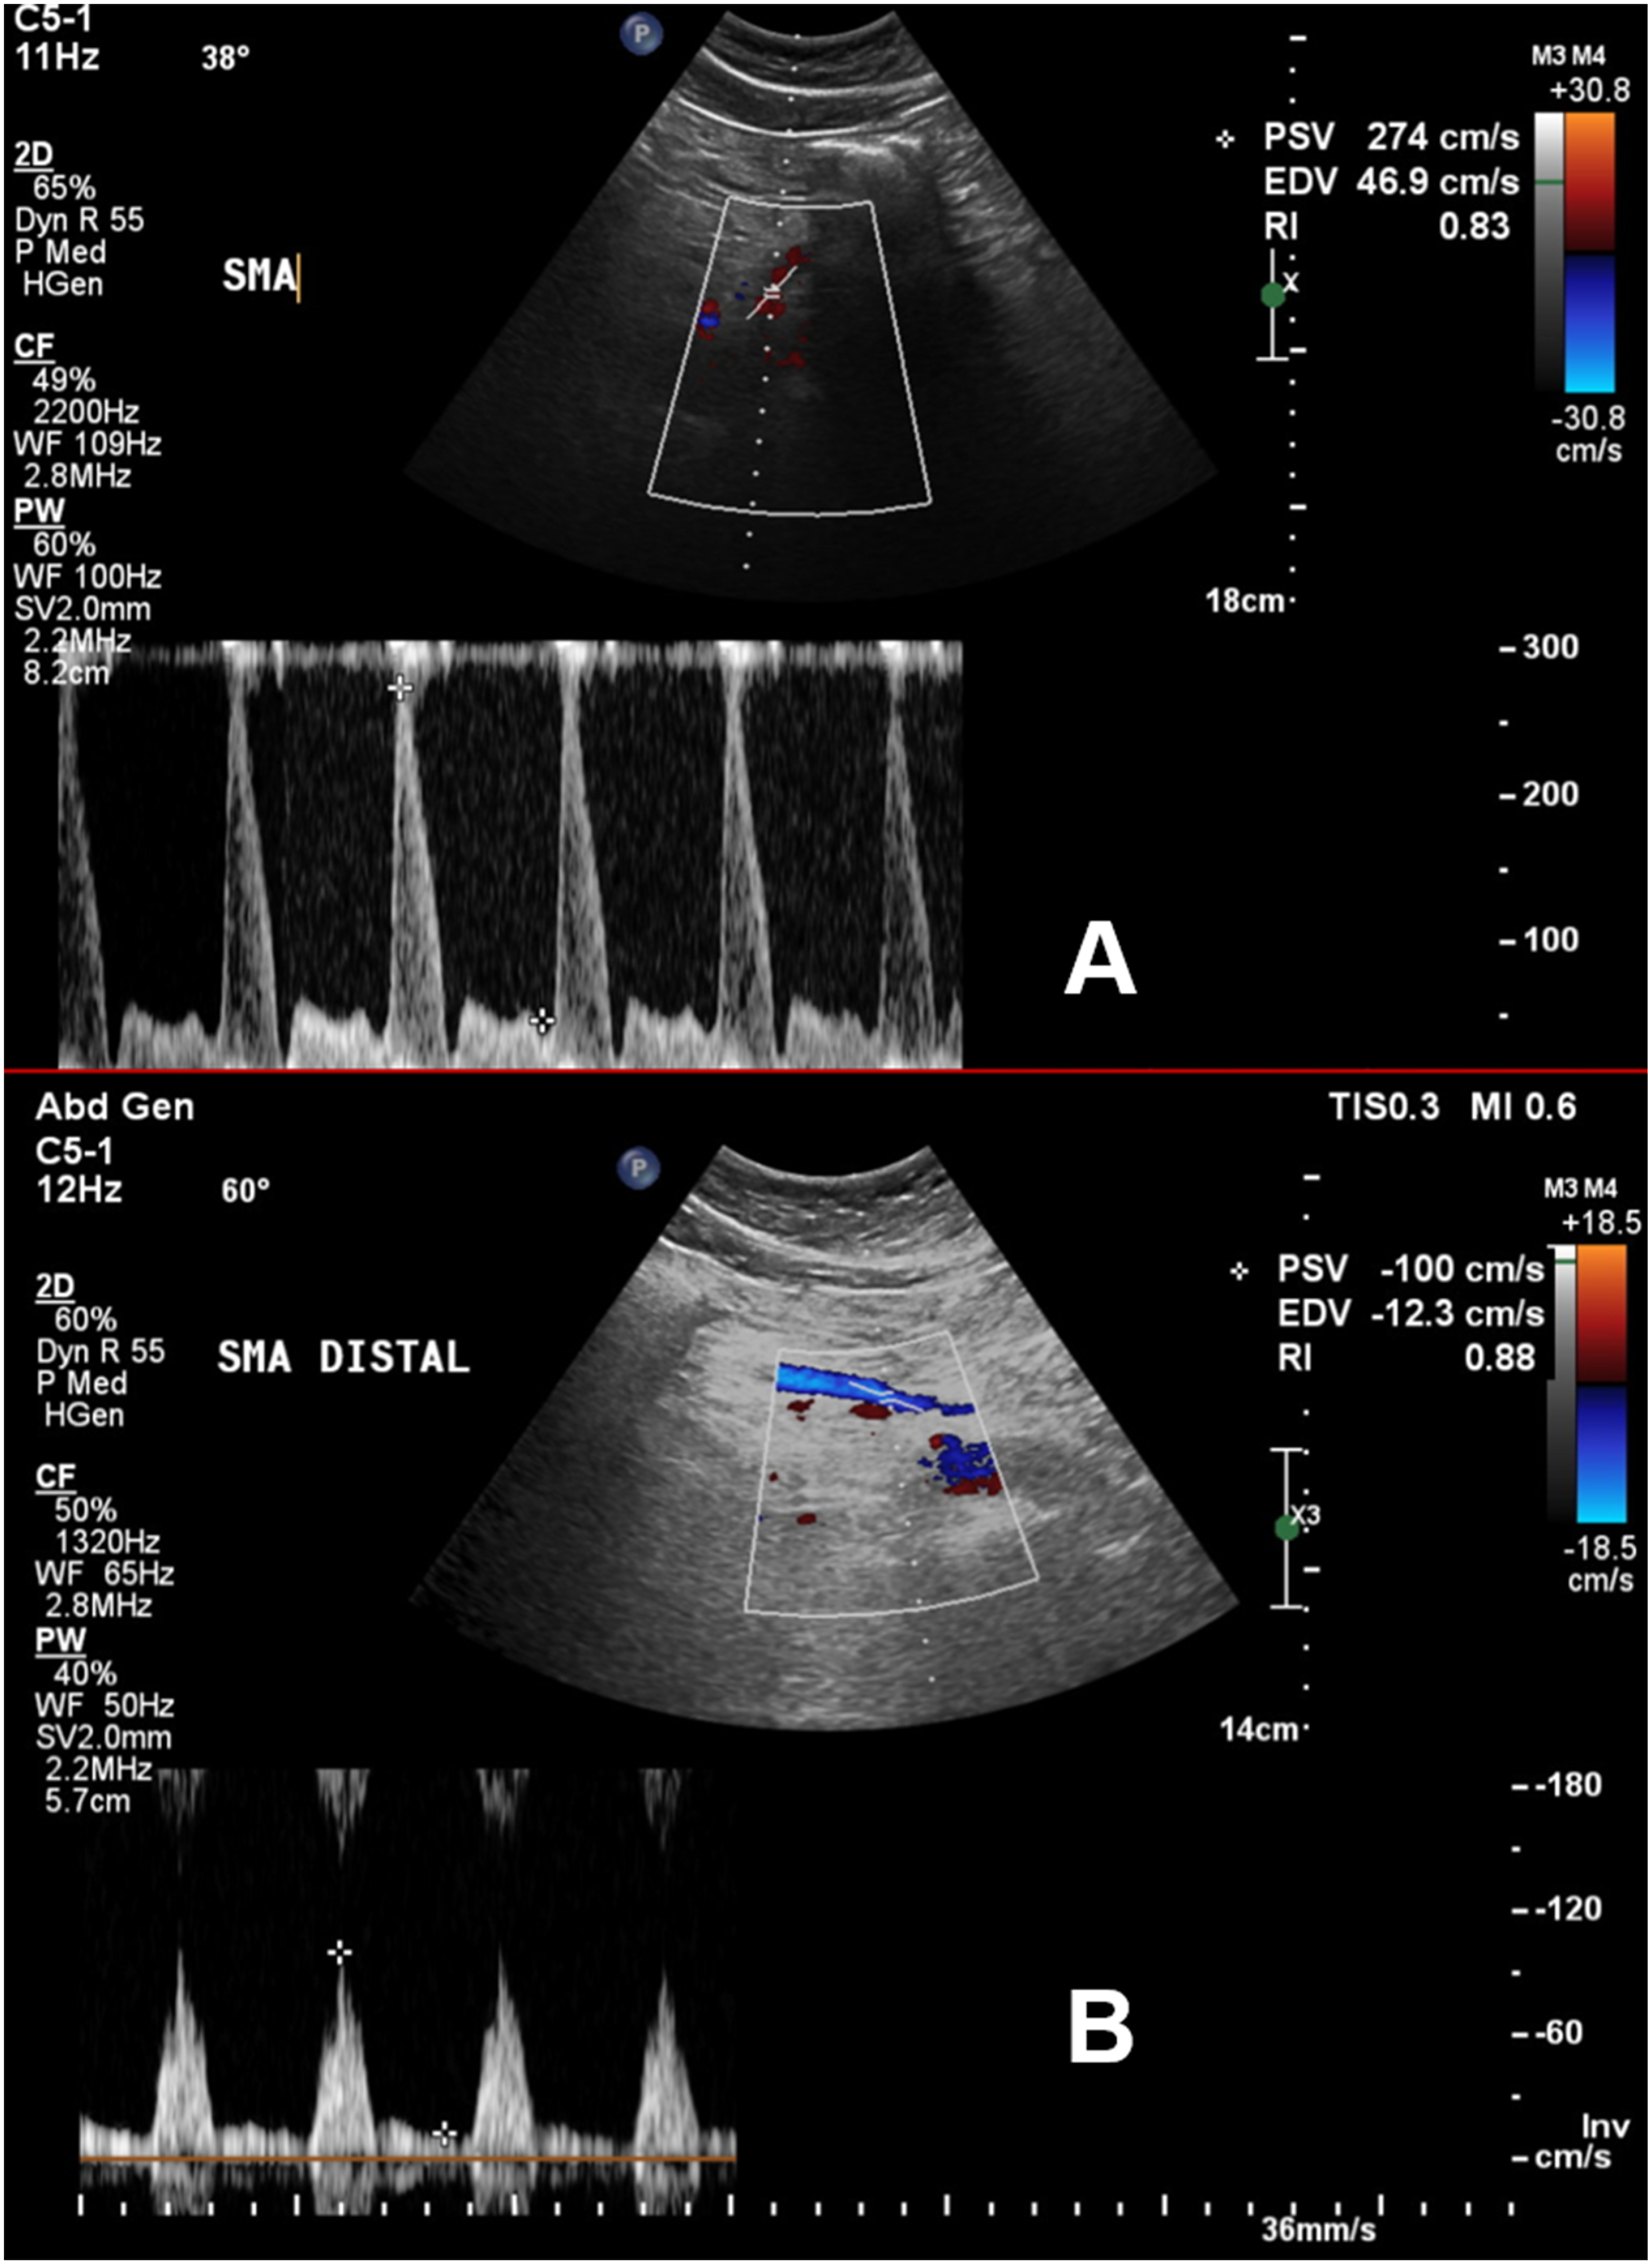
Fig. 4 (A) Duplex ultrasound 2 years post repair. There is increased velocity in the proximal superior mesenteric artery (SMA), indicating stent stenosis. (B) Duplex ultrasound 2 years post repair. The velocity is normal in the distal SMA, indicating patency and normal perfusion.
